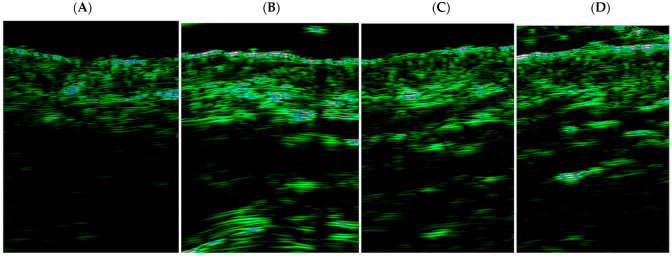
Ultrasound measurements of the forehead region—Patient #1: (A) before the treatment, (B) one month after 1st treatment, (C) one month after 2nd treatment, and (D) one month after the 3rd treatment. (The ultrasound images were acquired using a 22 MHz probe, visualizing skin structure. Green regions indicate areas of high echogenicity, representing dense structures such as the epidermis, dermis, and collagen fibers. Black regions correspond to areas of lower echogenicity, likely representing less dense connective tissue or deeper layers. Differences in green intensity reflect variations in skin thickness and density after treatment.).
